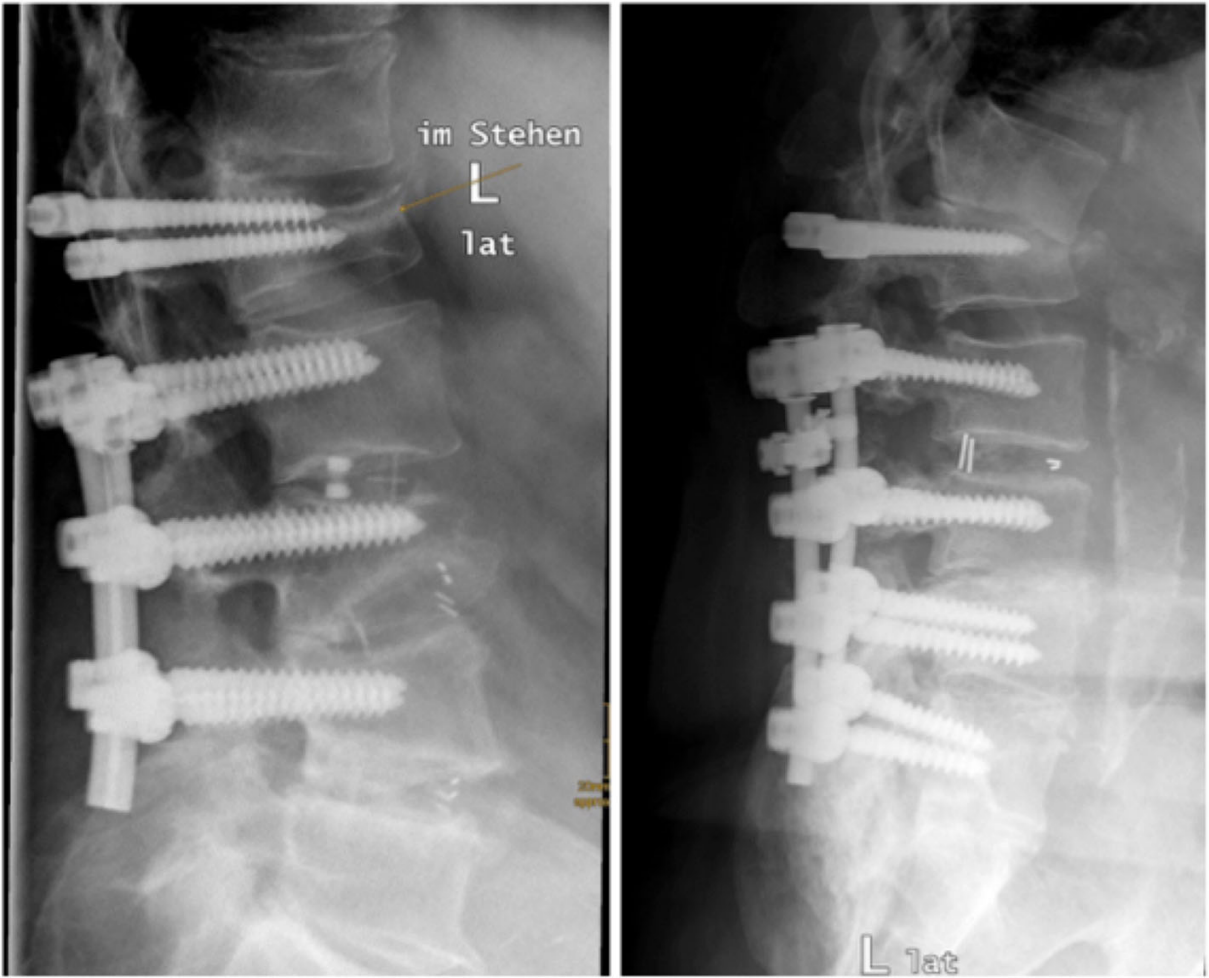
Radiographs in lateral view showed a vertebral fracture of the topping off vertebra (L1)
